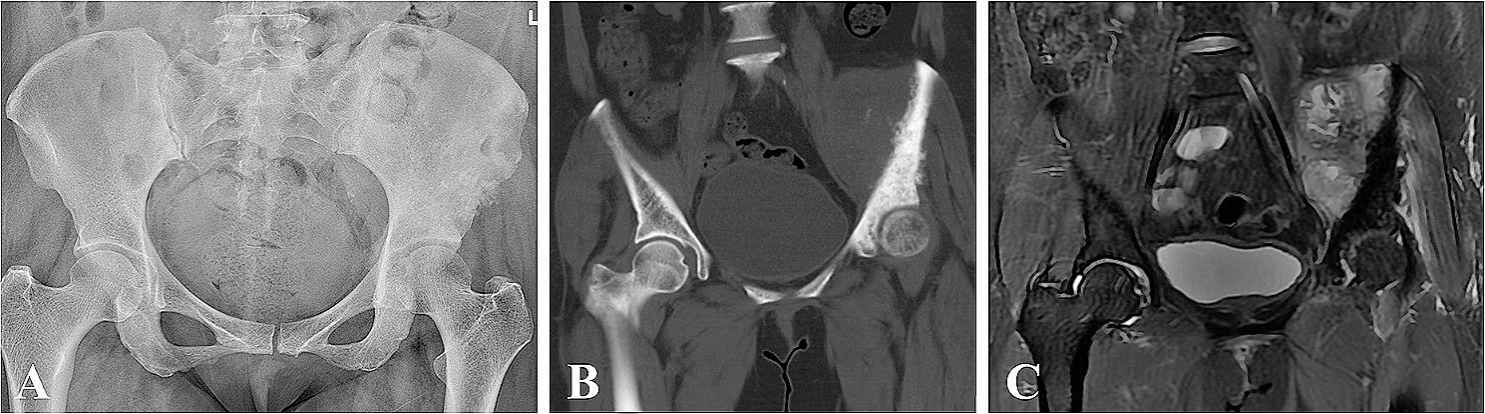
Preoperative X-ray (A), CT (B), and MRI (C) scans of a 50-year-old patient with osteosarcoma involving the acetabulum as well as most of the ilium
